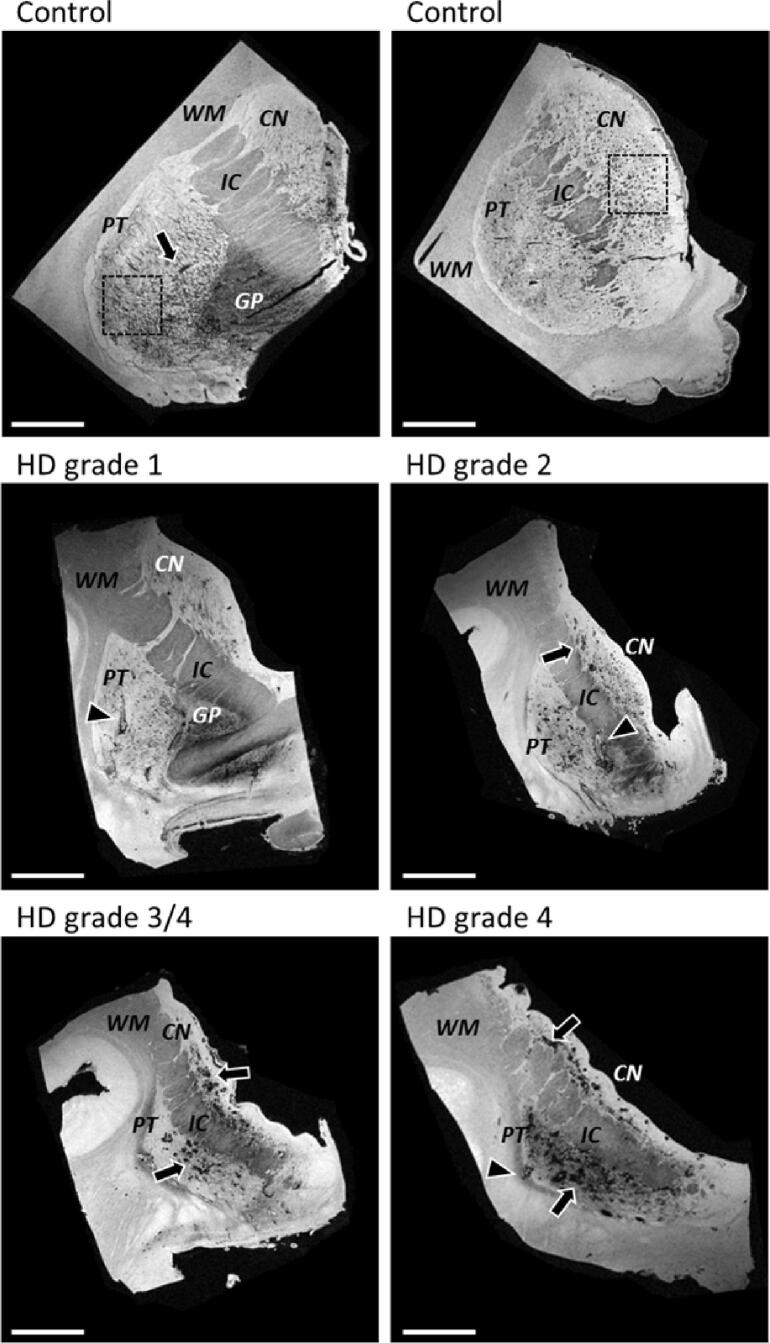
Representative images of T2*-weighted MRI of striatal tissue blocks of two controls and four HD cases. Gross examination of the HD patients showed a varying degree of atrophy which is also represented by the neuropathological severity as indicated by the Vonsattel grade. Small focal hypointensities were found within the caudate nucleus and putamen in control subjects (example within box, but also outside box present). In HD, both the caudate nucleus and putamen showed larger focal hypointensities, which were only rarely observed in control subjects. These larger focal hypointensities sometimes completely lacked any signal (arrow), and were sometimes filled with a hyperintense core probably as a result of residual PBS trapped within these spaces (arrow head). In general, the HD striata were atrophic and more hypointense compared to control striate in line with previously reported *in vivo* findings. CN = caudate nucleus; PT = putamen; IC = internal capsule; GP = globus pallidus; WM = white matter. Scale bar = 1 cm.
